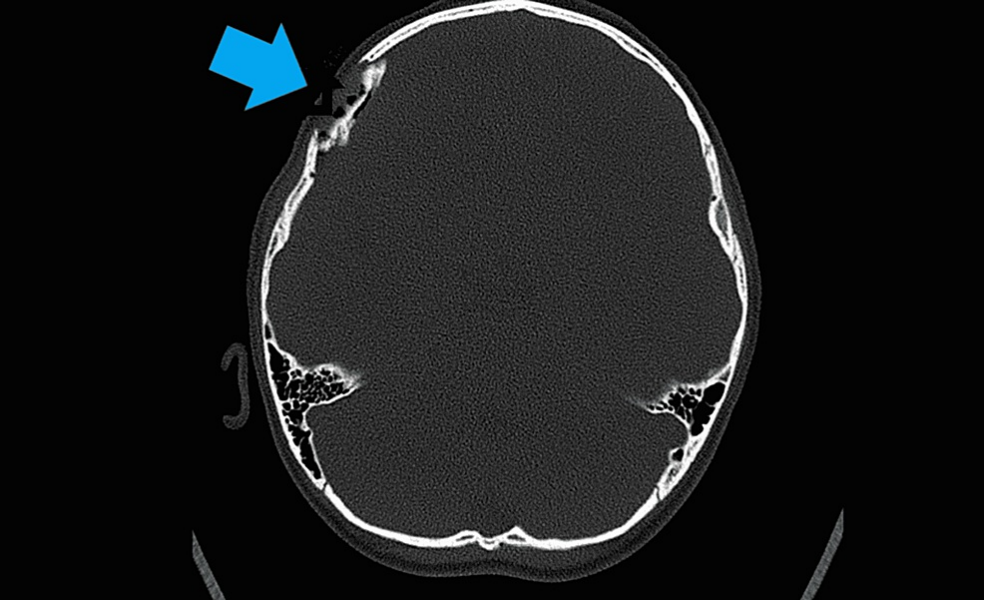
The blue arrow shows the right depressed frontal bone fracture with pneumocranium on the non-contrast computed tomography of the brain (bone window).
